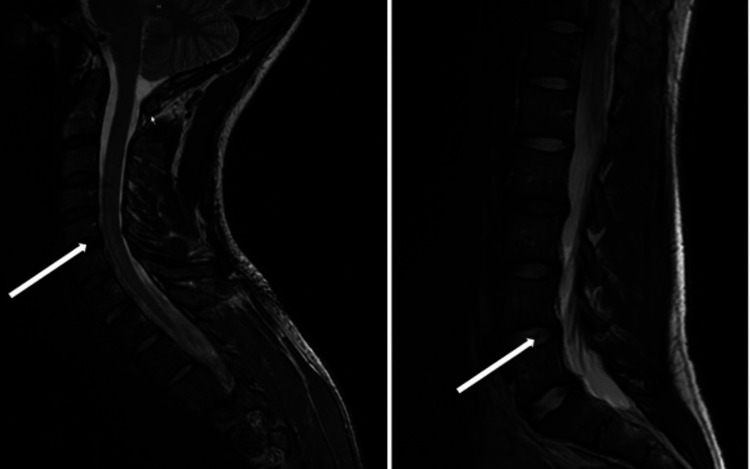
MRI of the cervical and lumbar spines indicated C5-C6, C7 slight bulging, and minor L4-L5 and L5-S1 disc herniation.
